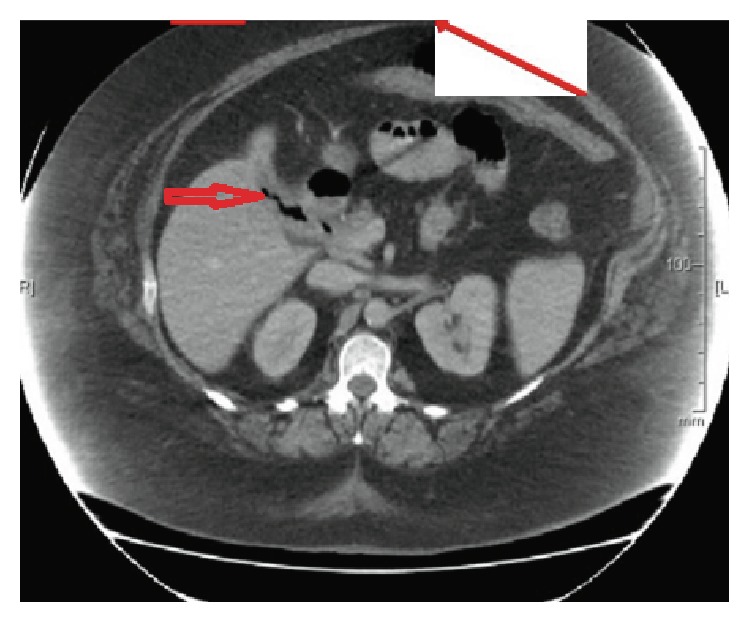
CT contrast scan depicting decompressed GB and air in GB, verifying that a stone was released (short red arrow).
